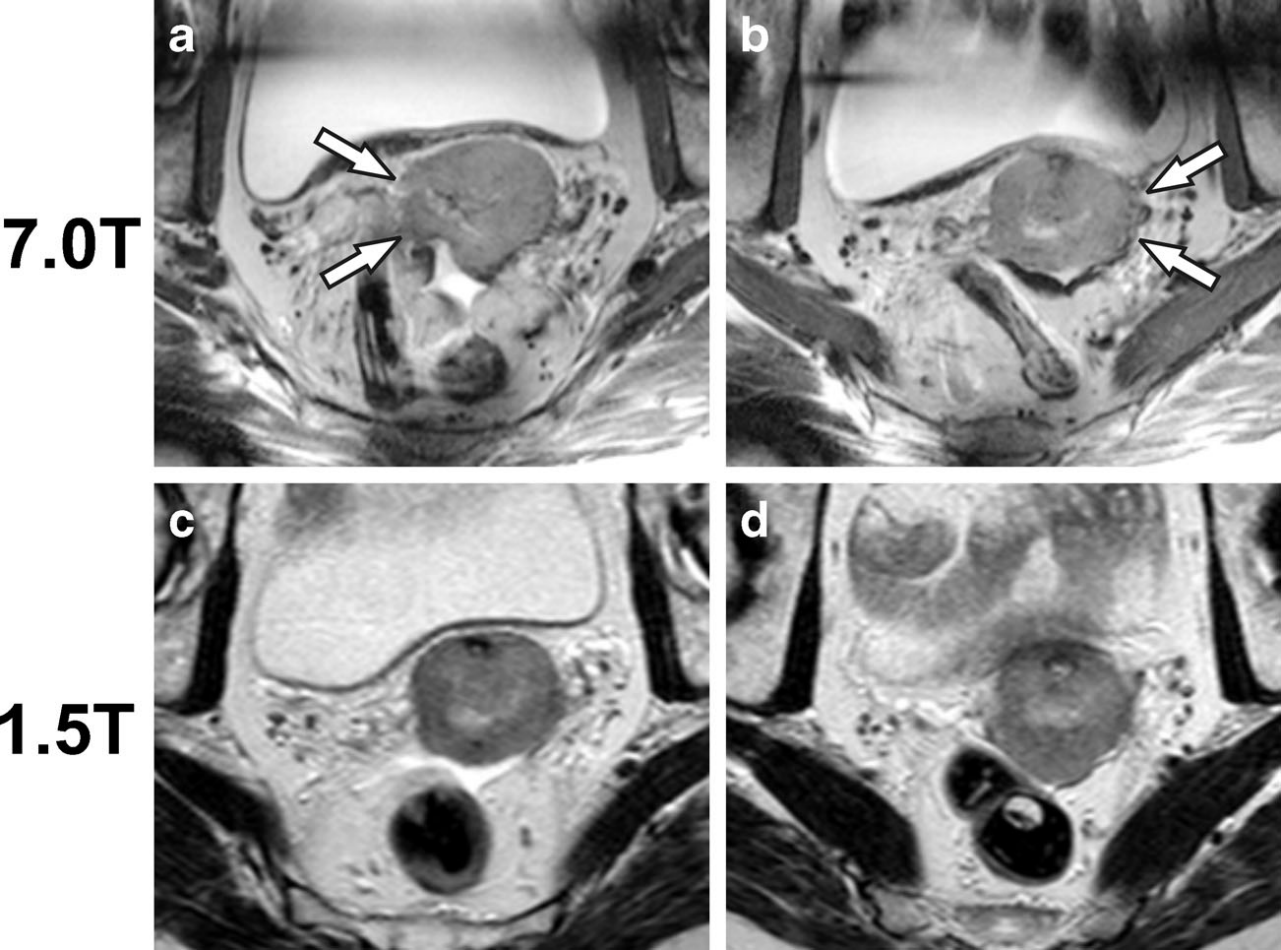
a Transverse T_2_-weighted acquisition from the 7.0-T MRI of a 65-year-old woman diagnosed with a 50-mm moderately differentiated squamous cell carcinoma of the cervix. b Slice from the same acquisition as a, though positioned 8 mm cranially. Only left-sided parametrial invasion was judged present at rectovaginal palpation, leading to a clinical stage IIB. However, the bilaterally unclear tumour demarcation and absent T_2_ hypointense fibrostromal ring are suggestive of bilaterally sided parametrial invasion (*arrows*). c, d The matched transverse T_2_-weighted slices from the clinical 1.5-T MRI, created 16 days earlier, are provided for comparison. Note the free fluid in the rectouterine pouch (Douglas)
